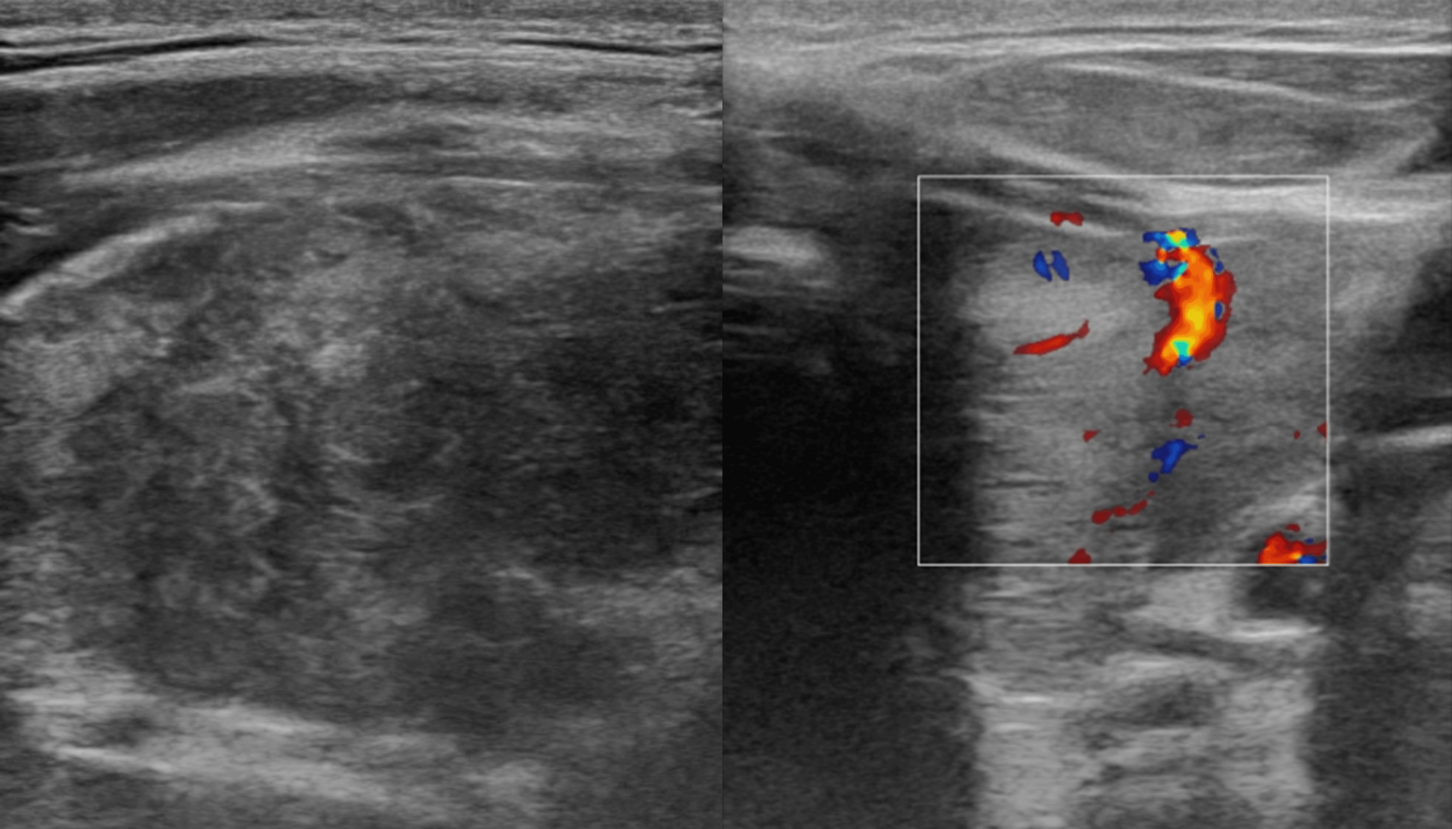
Thyroid ultrasound images Thyroid gland with heterogenous echotexture, geographic diffuse hypoechoic areas, and mildly increased parenchymal vascularity on color Doppler
